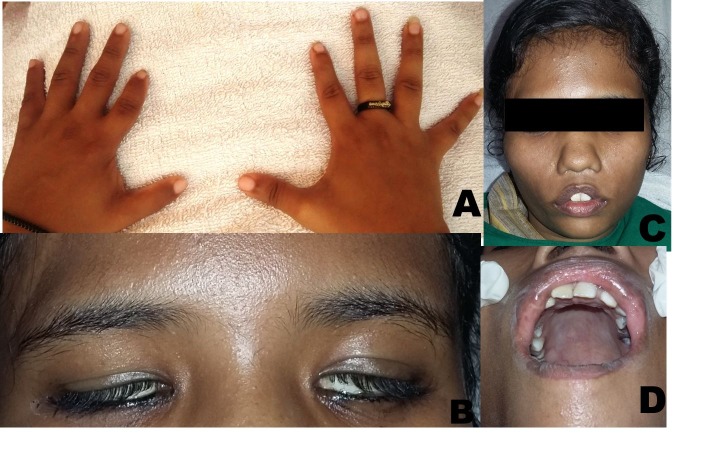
Based on history given by the patient and features shown on clinical examination, the case was diagnosed as Mobius syndrome. Routine hematological investigations were within normal limits. Panoramic view showed decays with respect to maxillary left second premolar, first molar and mandibular right and left first molar (Figure 2, A). Wrist radiographs showed short middle phalanges in the index finger of both hands (Figure 2, B and 2, C). A lateral cephalogram showed no abnormality (Figure 2, D). Correction of incompetent lips was planned using a multidisciplinary approach.
